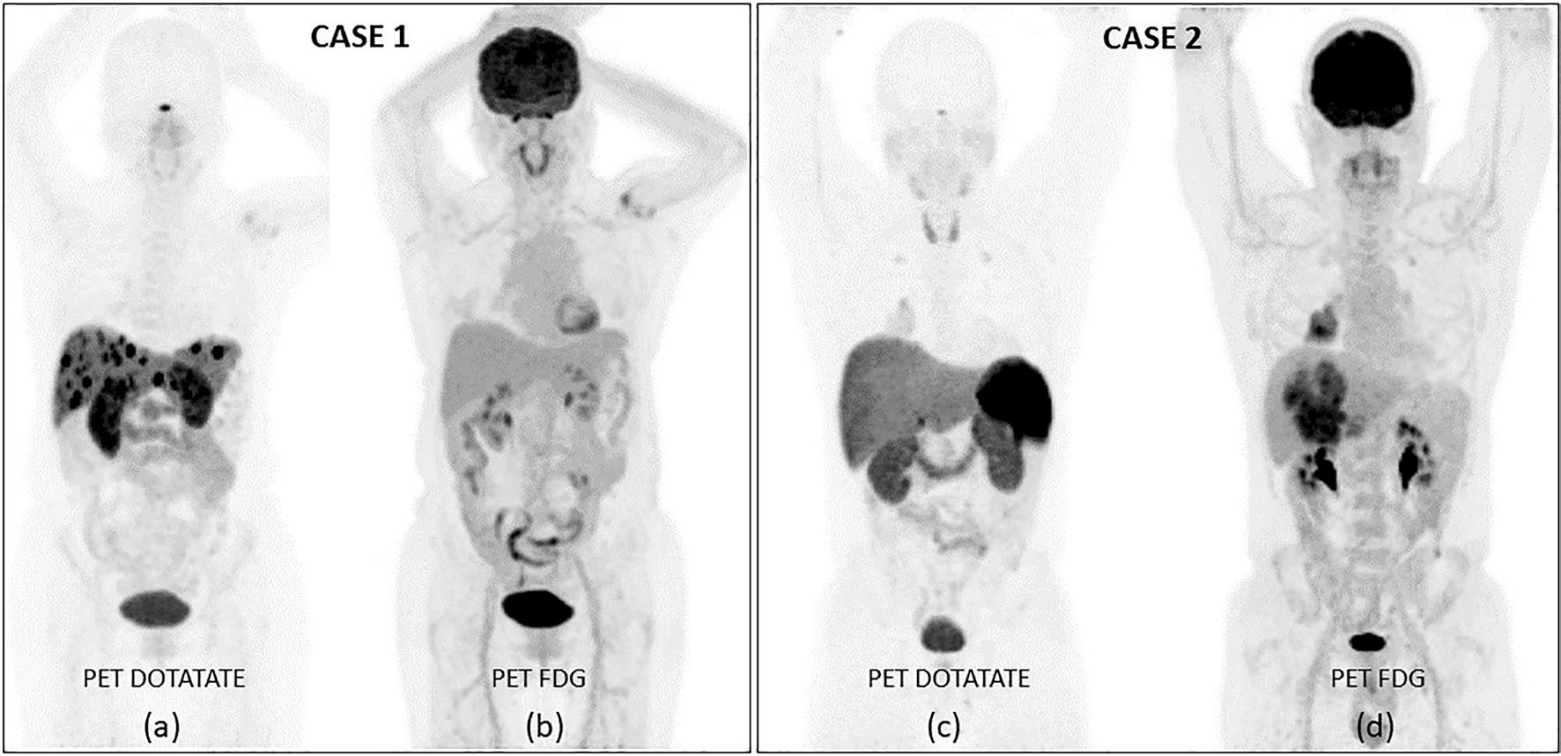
In case 1 (left), the a PET DOTATATE scan shows multiple hepatic SSTRI-positive lesions which have no uptake on the corresponding b PET FDG scan. The patient went on to successful treatment with LUTATE. In Case 2 (right), however, the c PET DOTATATE scan is essentially negative in the liver, while in d the PET FDG scan demonstrates significant uptake in both the lung lesion and the liver. This patient was deemed not suitable for treatment with LUTATE and was offered systemic medical therapy instead
